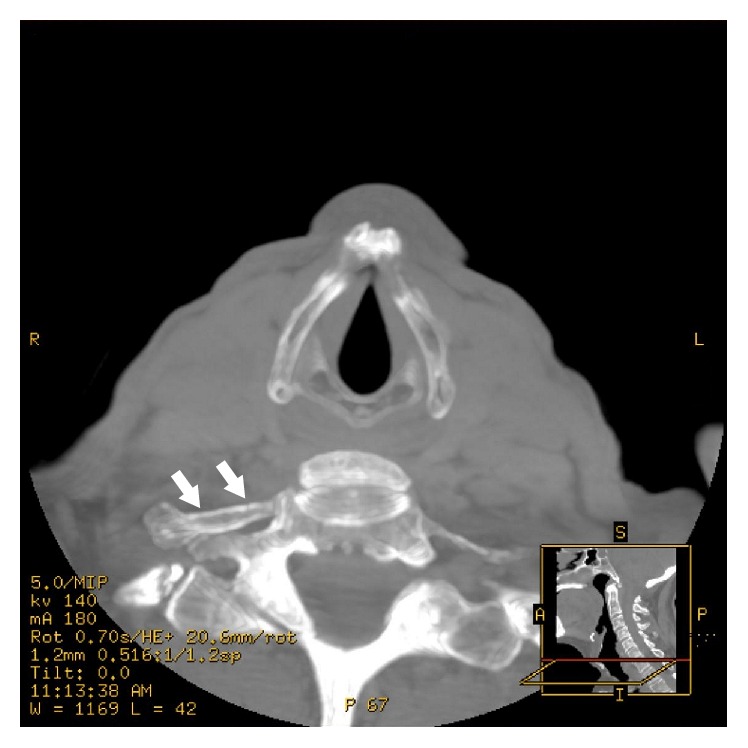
A computed tomography transverse (CT) scan at the level of the seventh cervical vertebra demonstrating unilateral cervical rib (arrows).
